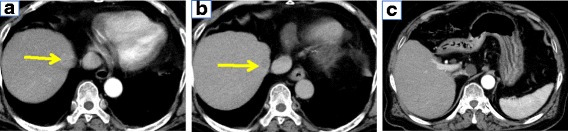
CT shows the agenesis of the left hepatic lobe accompanied by HCC. a Arterial phase of dynamic CT shows a 15-mm hypervascular mass in segment 8. b Delayed phase shows a hypovascular mass. c Abdominal CT shows the nonexistence of the left hepatic lobe
